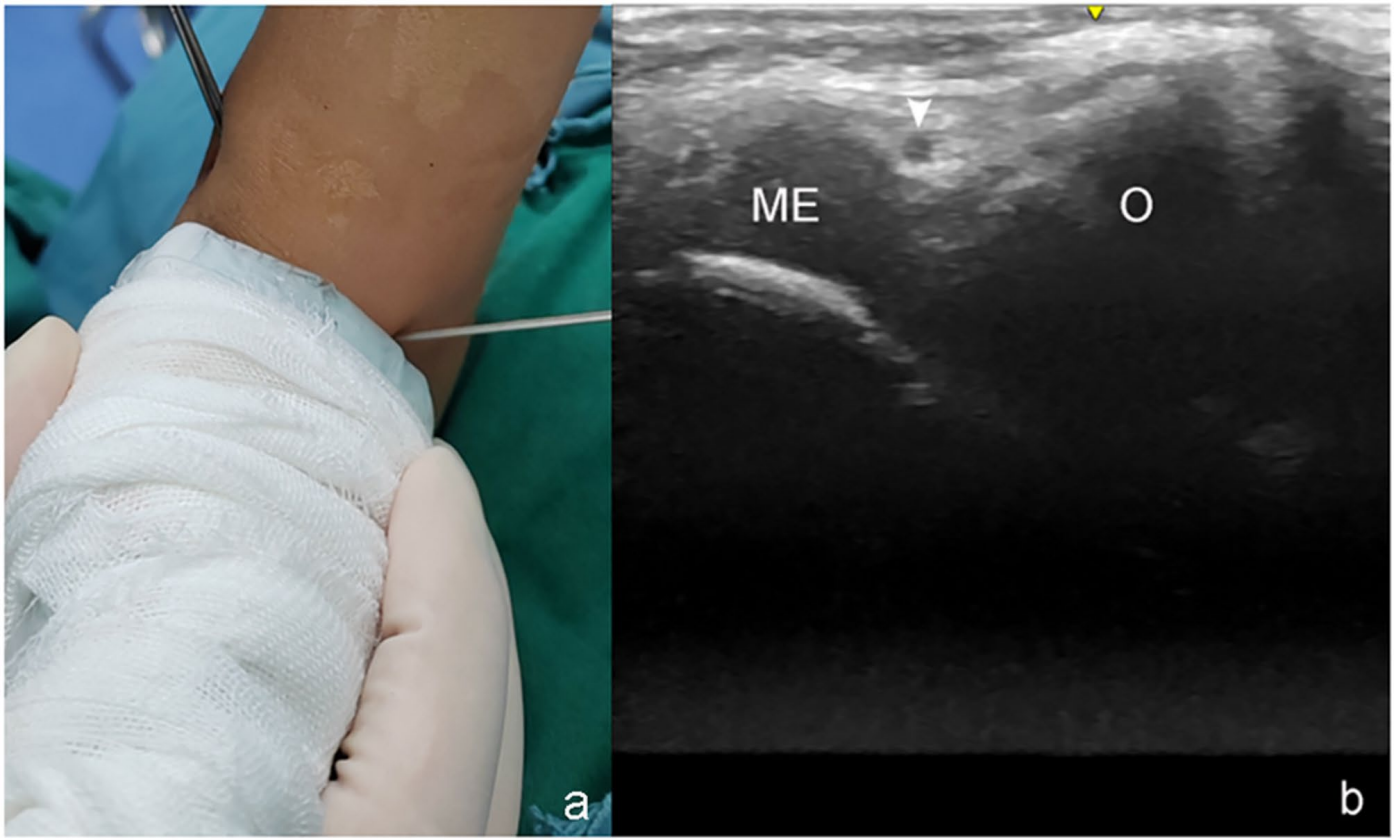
Photographs showing the positions of the transverse transducer (a), and corresponding ultrasound sections (b). The ultrasound reveals characteristic hypoechoic ulnar nerve (arrowhead), and the hypoechoic border of the medial epicondyle and olecranon with a typical acoustic shadow from the transverse sections. ME: Medial epicondyle of humerus; O: olecranon
